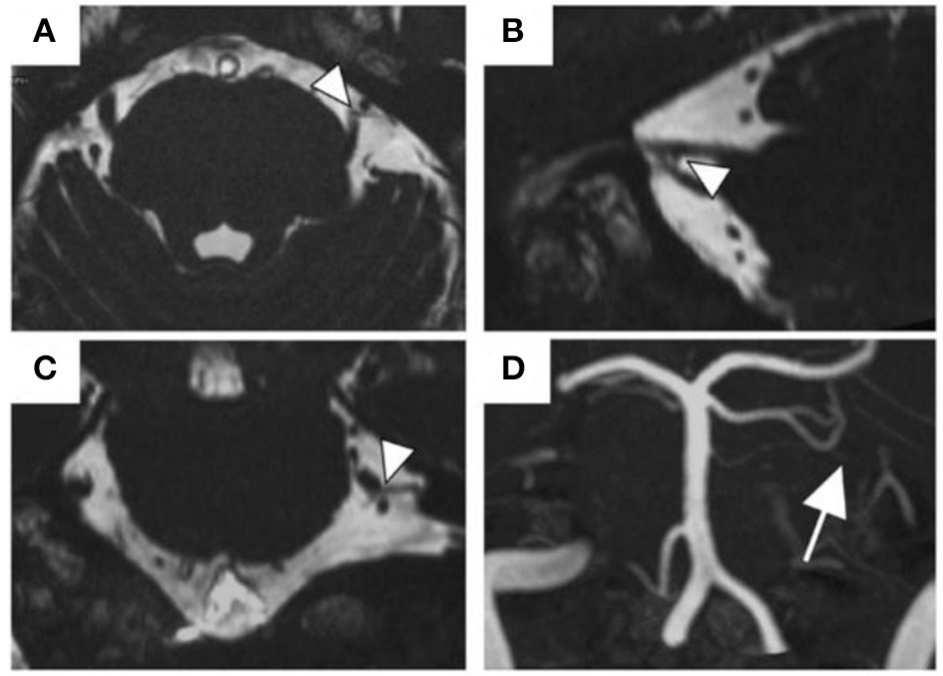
The MR images of NVC of the trigeminal nerves. A 57-year old man with NVC of the trigeminal nerves (arrowheads). The superior cerebellar artery was the responsible blood vessel (arrow). (A) Transverse view of the 3D-CISS image, (B) sagittal view, (C) coronal view, (D) MIP display of 3D-TOF MRA. Abbreviations: MR, magnetic resonance; NVC, neurovascular contact; 3D-CISS, 3D constructive interference in steady-stat; MIP, maximum intensity projection; 3D-TOF MRA, 3D time-of-flight magnetic resonance angiography.
